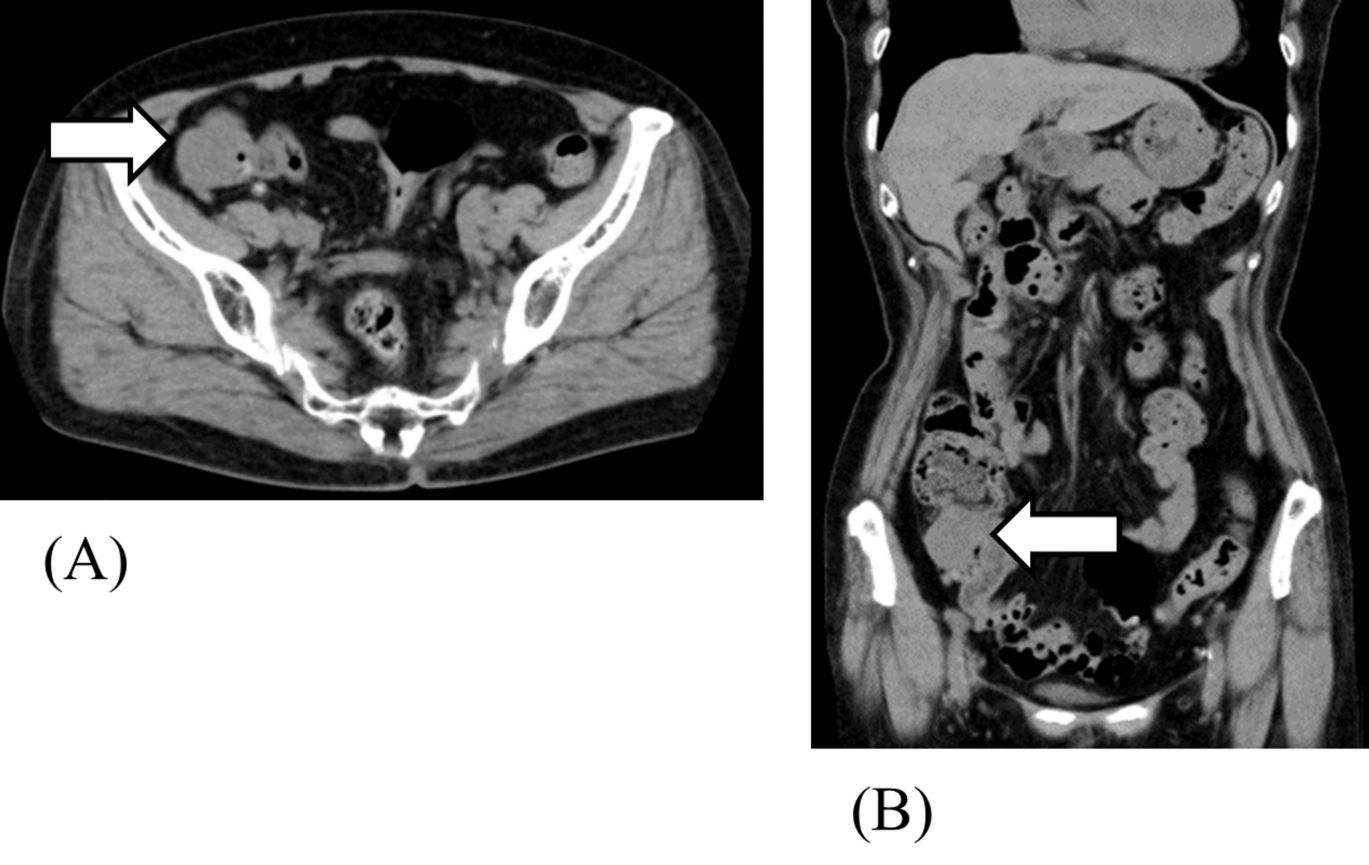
Abdominal plain CT findings obtained seven years earlier. Axial (A) and coronal (B) slice CT scans of the abdomen show that a known 3-cm submucosal mass is observed at the ileocecal region (arrow). No target sign is present at that time.
